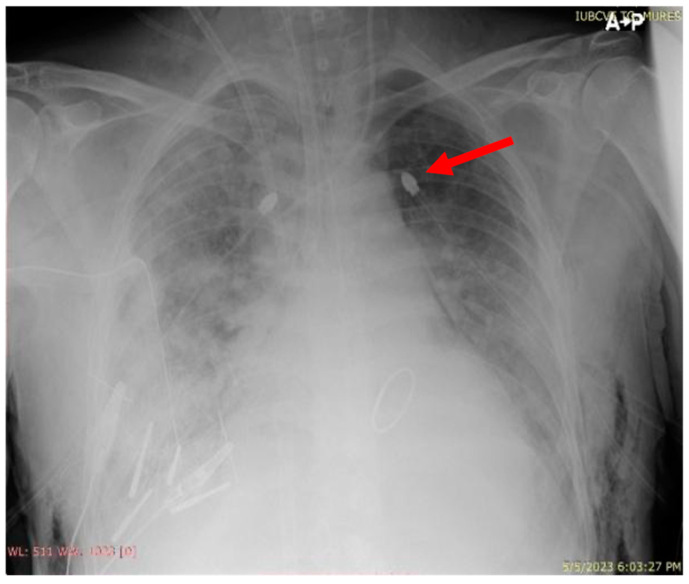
Thoracic X-ray after drainage (red arrow indicates drainage tube).
